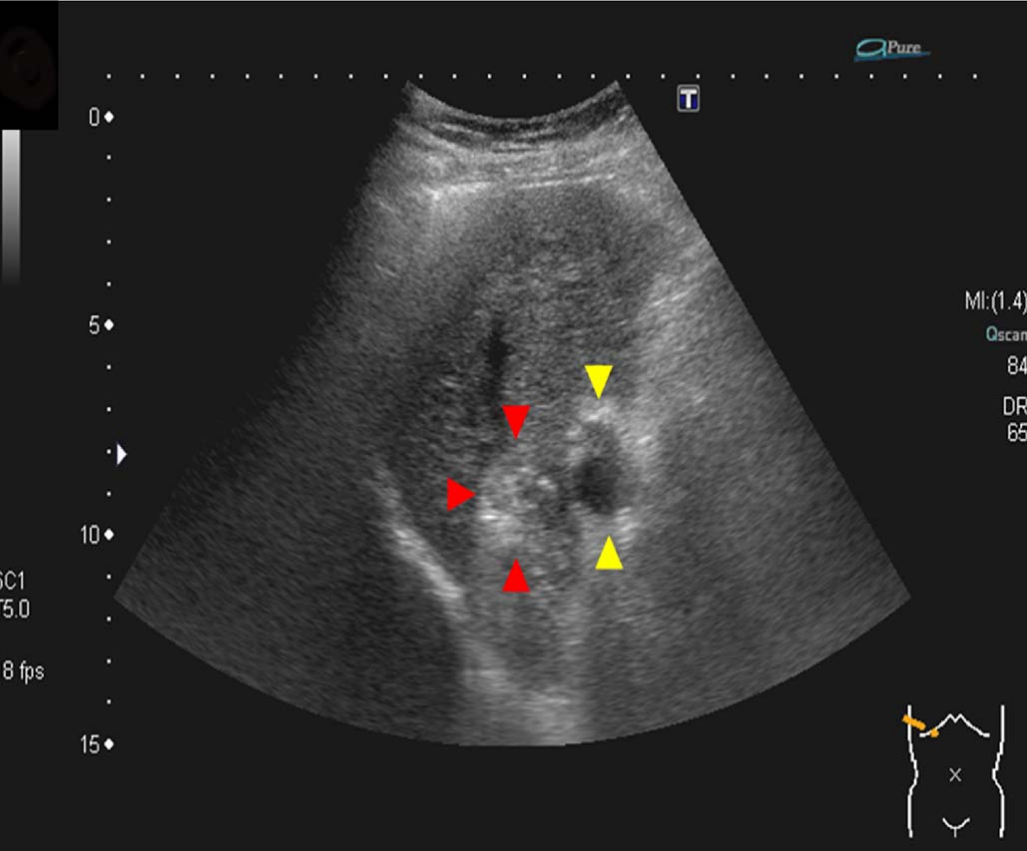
Ultrasound confirming enhancement of the bile duct (yellow arrowheads) adjacent to the hepatocellular carcinoma nodule (red arrowheads).
